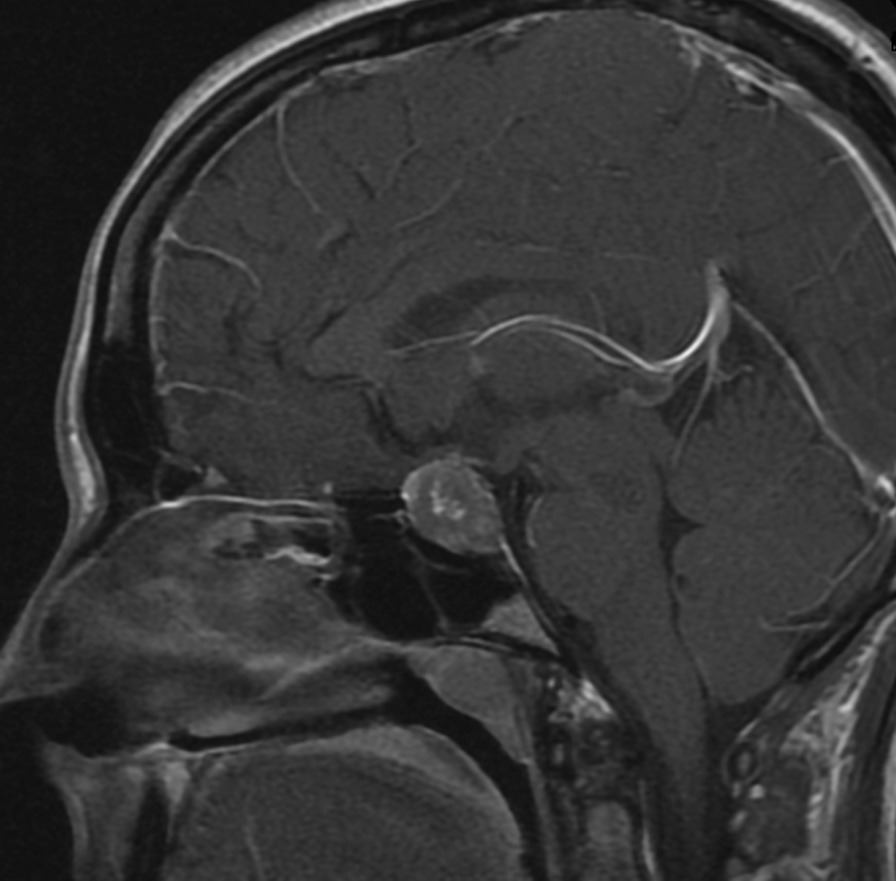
Magnetic resonance imaging showing pituitary macroadenoma with suprasellar extension and optic chiasm compression
